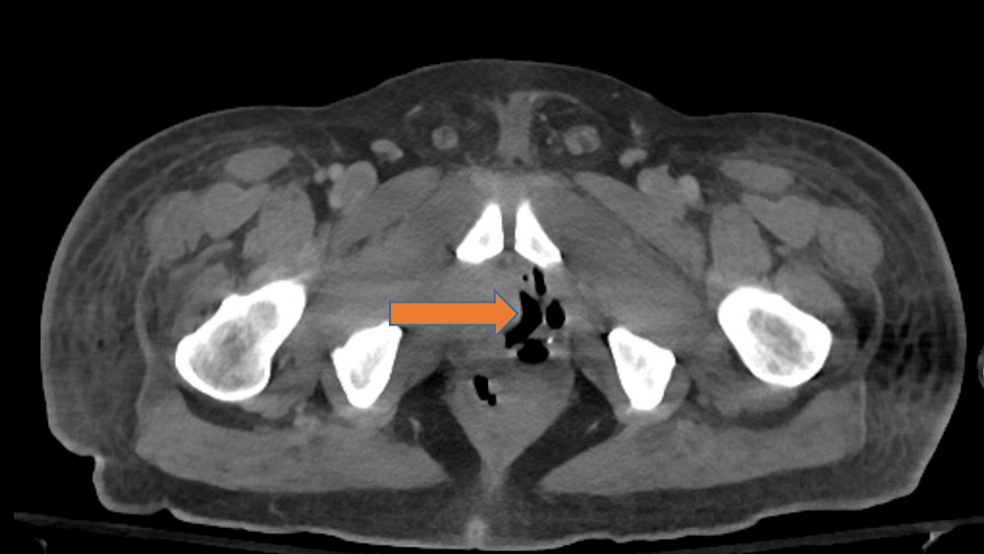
CT abdomen and pelvis showing enlargement and heterogeneity of the left seminal vesicles, with soft tissue fullness and obliteration of the fat planes between the rectum, prostate gland, and seminal vesicles(shown by orange arrow). These findings are concerning for gas-forming infectious process.
